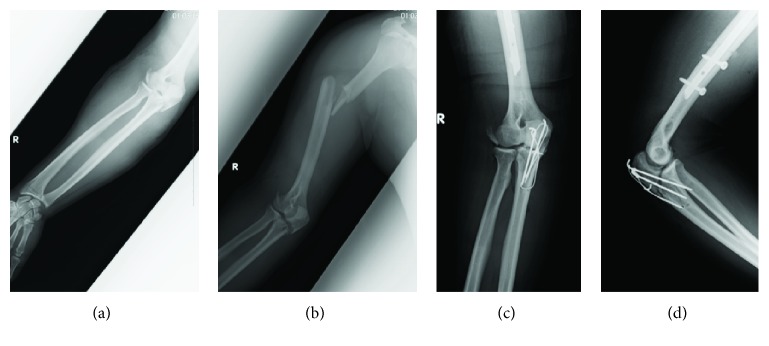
Radiographs of the injury and first surgery at the previous hospital. (a) Anteroposterior (AP) view of the forearm and elbow. (b) AP view of the humerus and elbow. Diaphyseal fracture of humerus, olecranon fracture, and lateral epicondylar fracture are found. (c) AP view and (d) lateral view of the elbow after surgery. The olecranon is fixed by tension band wiring. The lateral epicondylar fracture is displaced with normal joint congruence.
